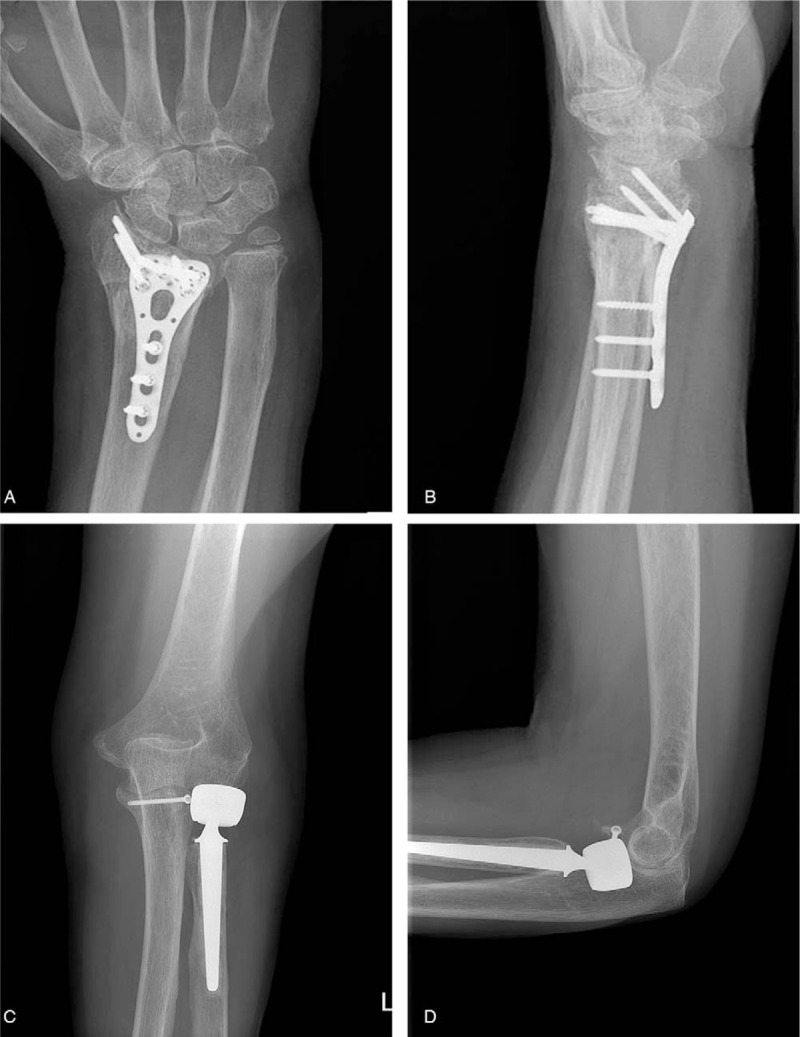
Postoperative plain radiographs of the wrist (A, B) and the elbow (C, D) of case 1 show that open reduction and internal fixation was performed for the distal radius and coronoid process fractures, and radial head replacement for the radial head fracture.
